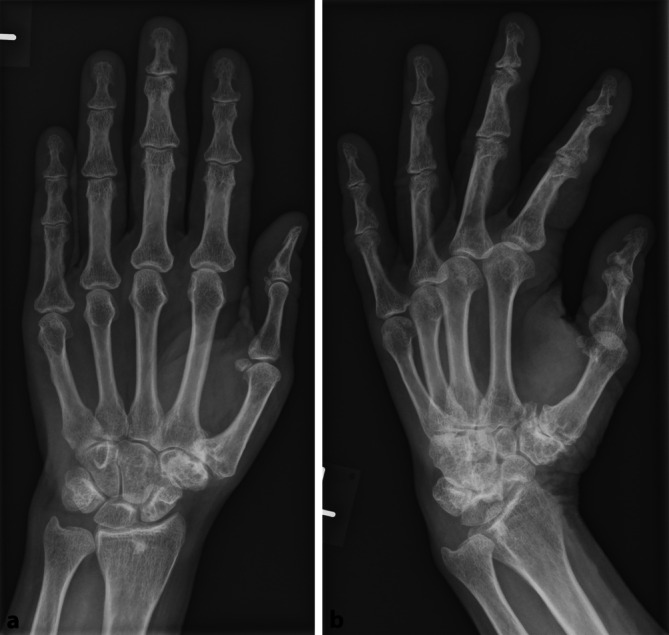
Das medulläre Osteom ist, wie auch ein Stein, charakteristischerweise scharf begrenzt. Anders als ein Stein besitzt es allerdings füßchenartige Ausläufer (Pseudopodien, [1]), die allerdings so fein sein können, dass sie im konventionellen Röntgenbild nicht sichtbar sind. Eine sichere Unterscheidung ist in diesem Fall aufgrund der auch zum intramedullären Osteom passenden Lage und Konfiguration des Steins nur durch die Dichtemessung einer CT-Untersuchung möglich. Als weitere Differenzialdiagnose für eine scharf begrenzte, röntgendichte Struktur gilt noch die osteoblastische Metastase, welche allerdings in diesem Fall aufgrund des Patientenalters nicht infrage kommt.
